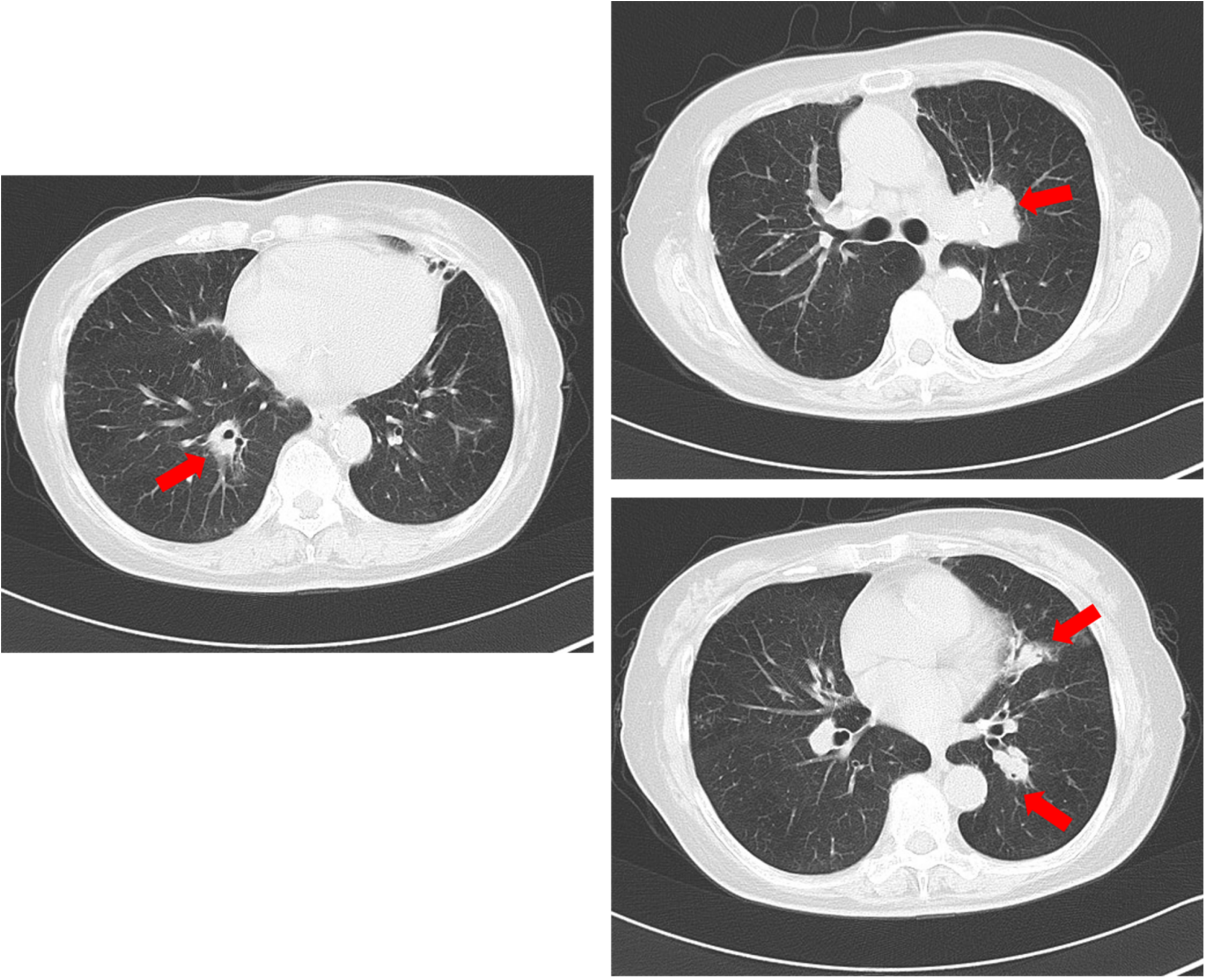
Chest computed tomography scan showing a 35‐mm nodule in her left S1 + 2 contiguous with the lymph nodes (A) and nodules around the right B10 (B), left B5 and B10a (C).
